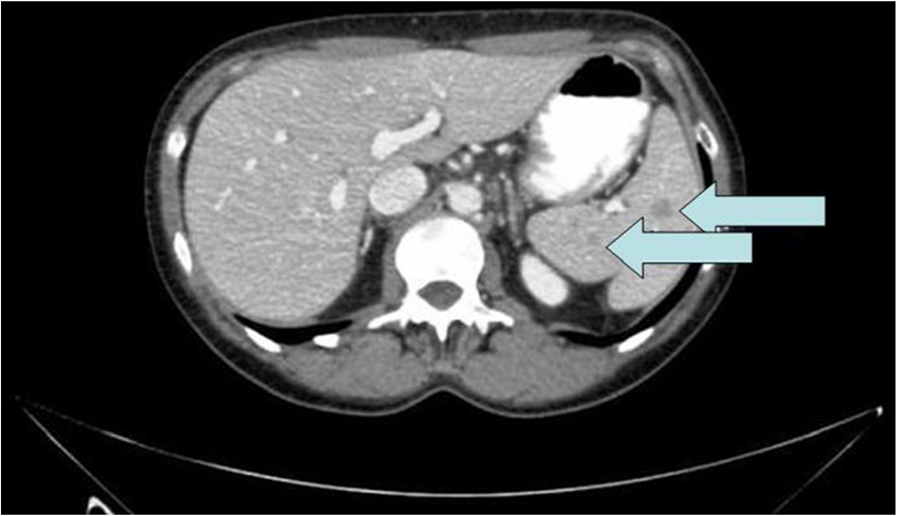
Dysmorphic and inhomogeneous spleen, with nodular millimeter areas – indicated with the arrows - which appeared hypodense during arterial and portal phases of the contrastographic study (transverse diameter max 11mm at abdominal CT scan)
